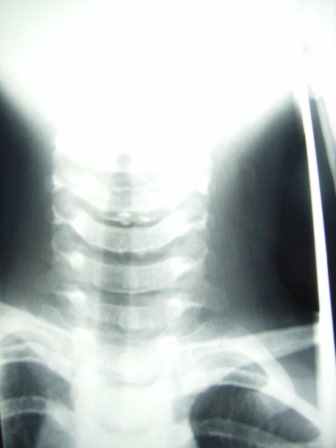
Calcifications inter discales à hauteur de C3 sur l’incidence de radiographie de face chez le patient 2
